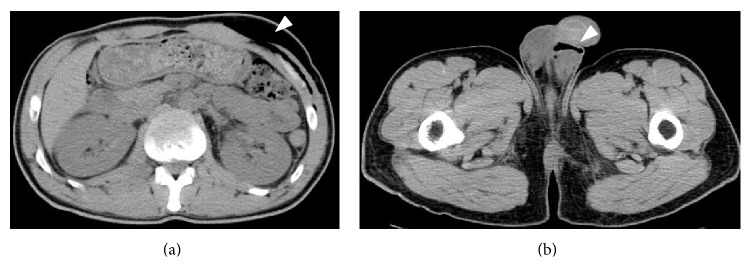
Abdominal CT after 10 days in the hospital. Subcutaneous gas remains in the left flank despite prompt disappearance of emphysema in the scrotum. Subcutaneous gas (arrow head).
